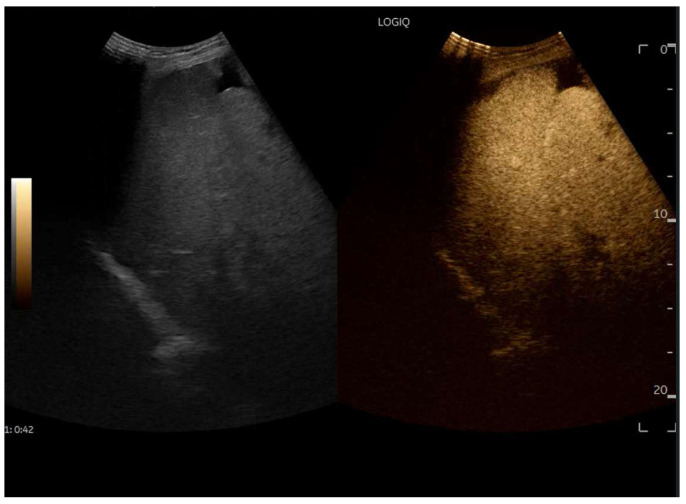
Contrast-enhanced ultrasound (CEUS) in the portal phase; The enhancement of the different hepatic regions becomes homogeneous.
